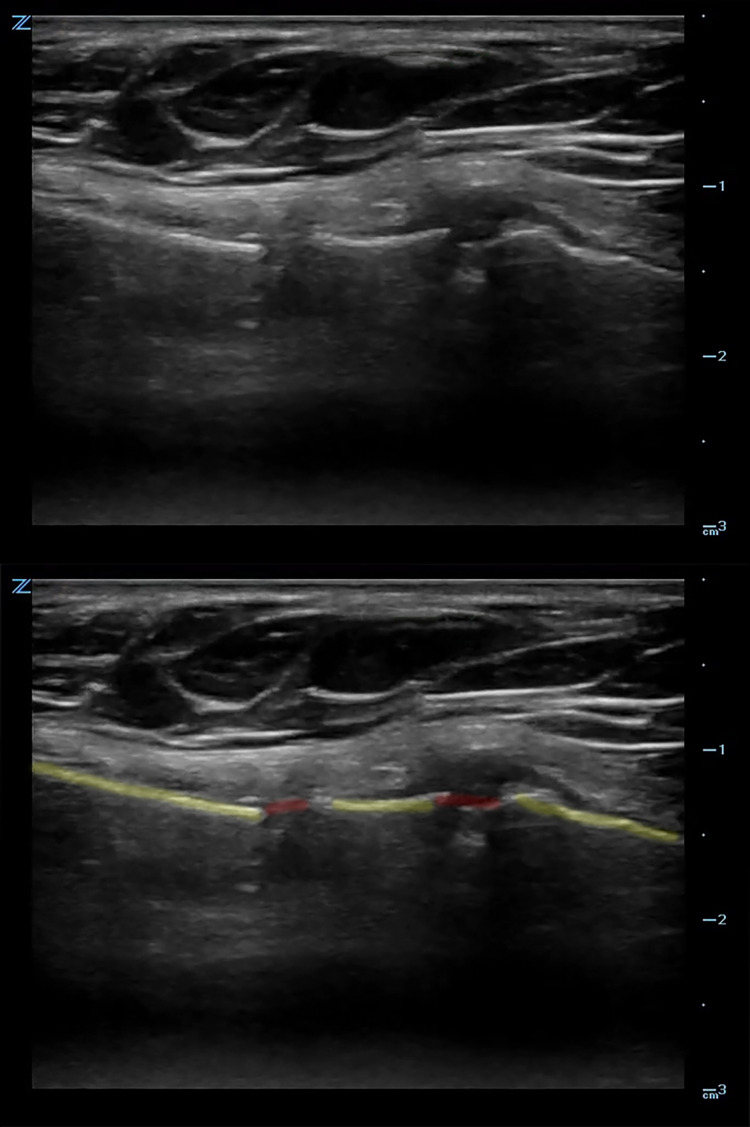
Ultrasound image of two areas of cortical disruption visualized on the sternum with overlying hematoma. The anterior cortex of the sternum (yellow) and the fracture sites (red).
